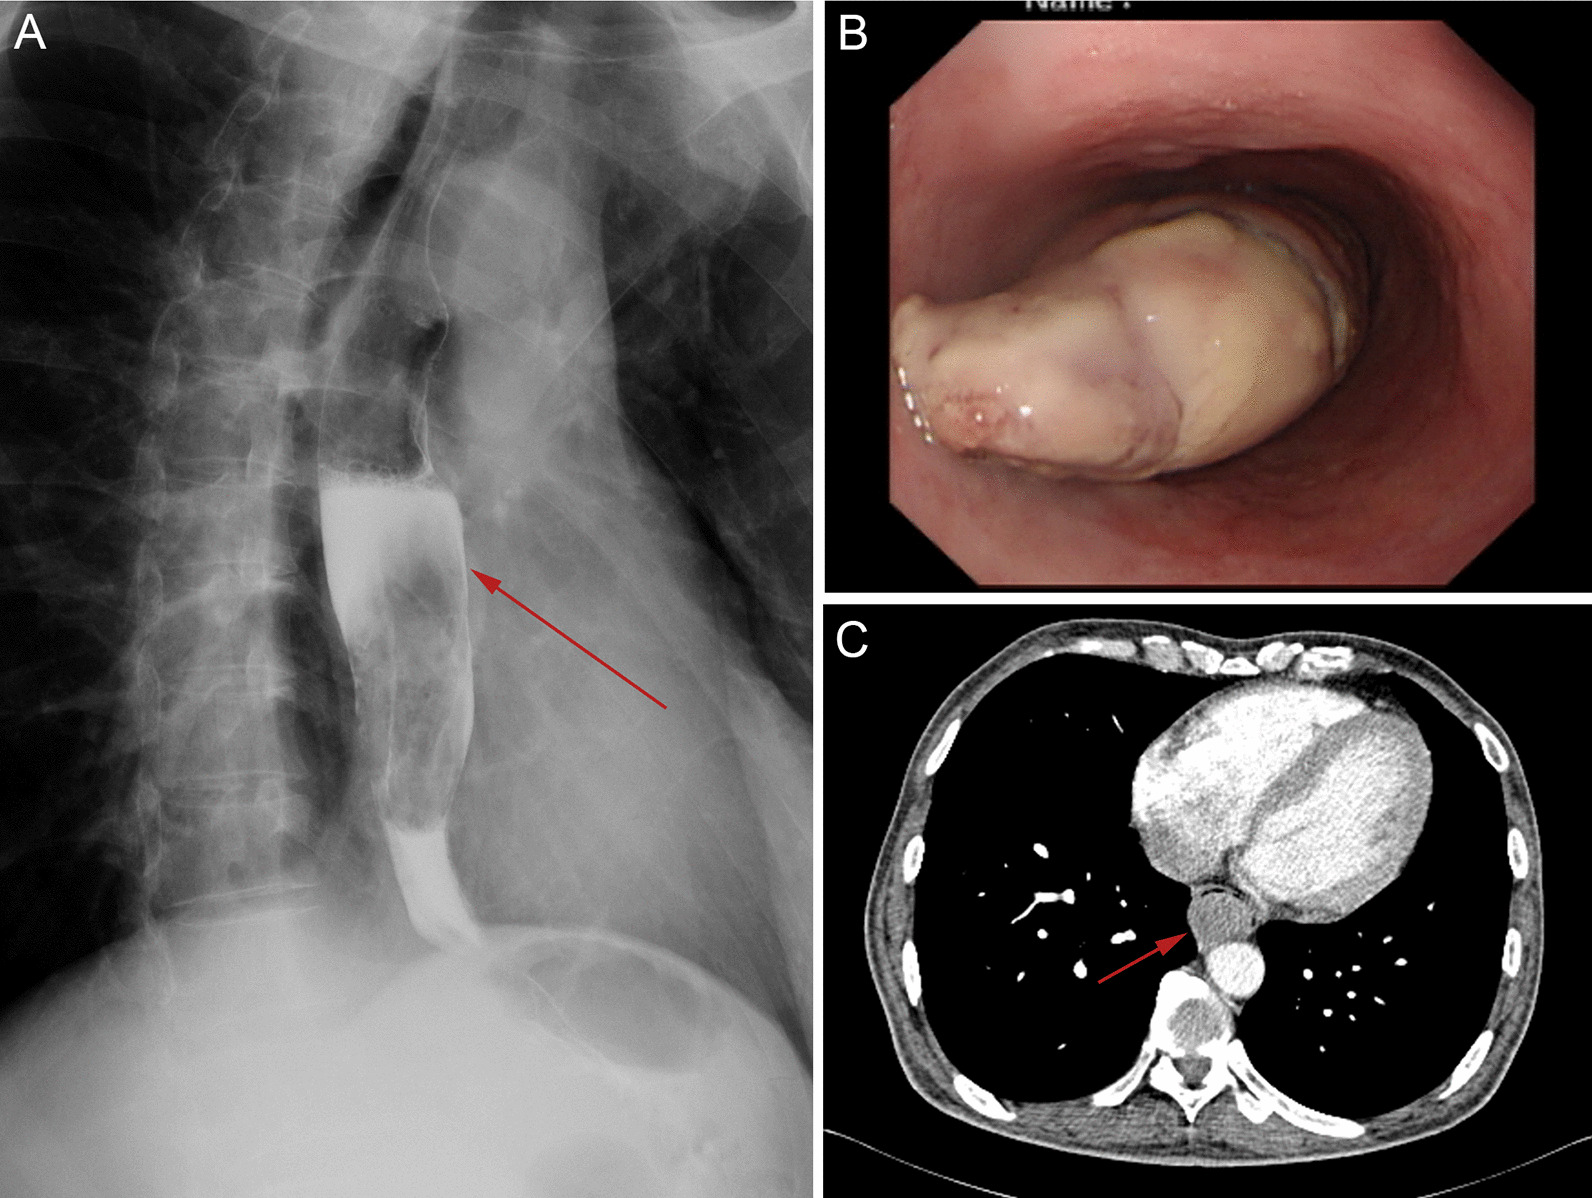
Radiographic and endoscopic examination of the mass. A X-ray examination shows an irregular approximately circular filling defect. B Endoscopic examination showed a gray-white mass in the esophageal lumen that was connected to the esophageal wall via a pedicle. C Contrast-enhanced computed tomography revealed a large mass obstructing the esophageal lumen without enlarged lymph nodes or invasion to the adjacent organs
